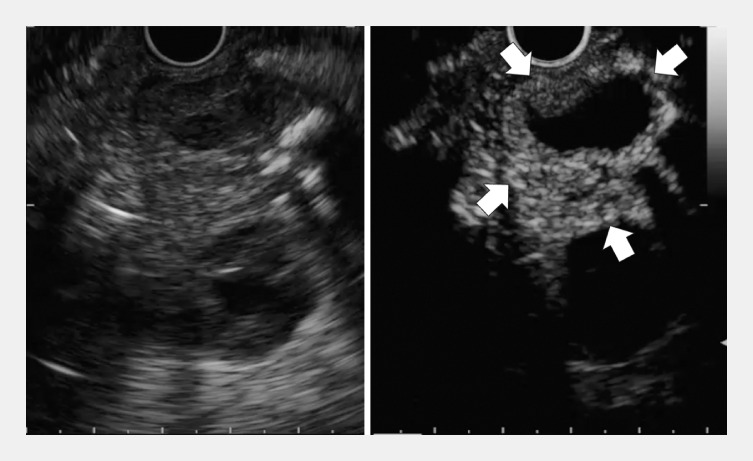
Contrast-enhanced endoscopic ultrasound images showing the 20-mm tumor (arrows), which was connected with the gastric wall.
